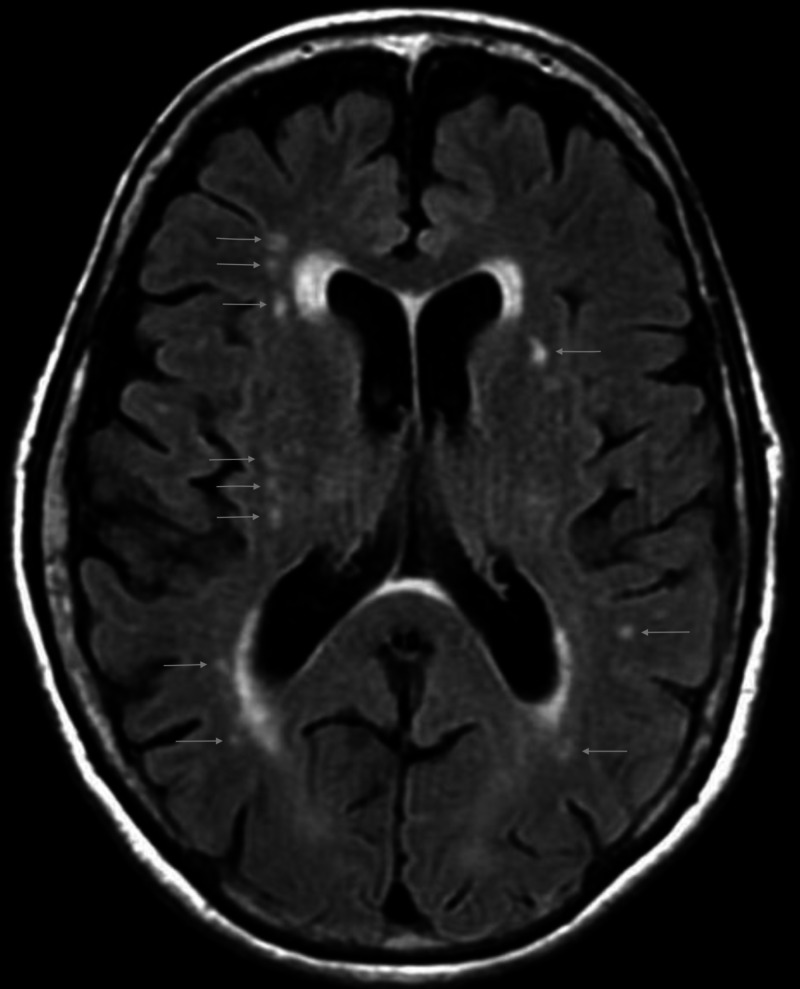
Multiple T2 hyperintense foci can be seen in the white matter on axial FLAIR consistent with chronic microvascular ischemic changes. FLAIR, fluid-attenuated inversion recovery
